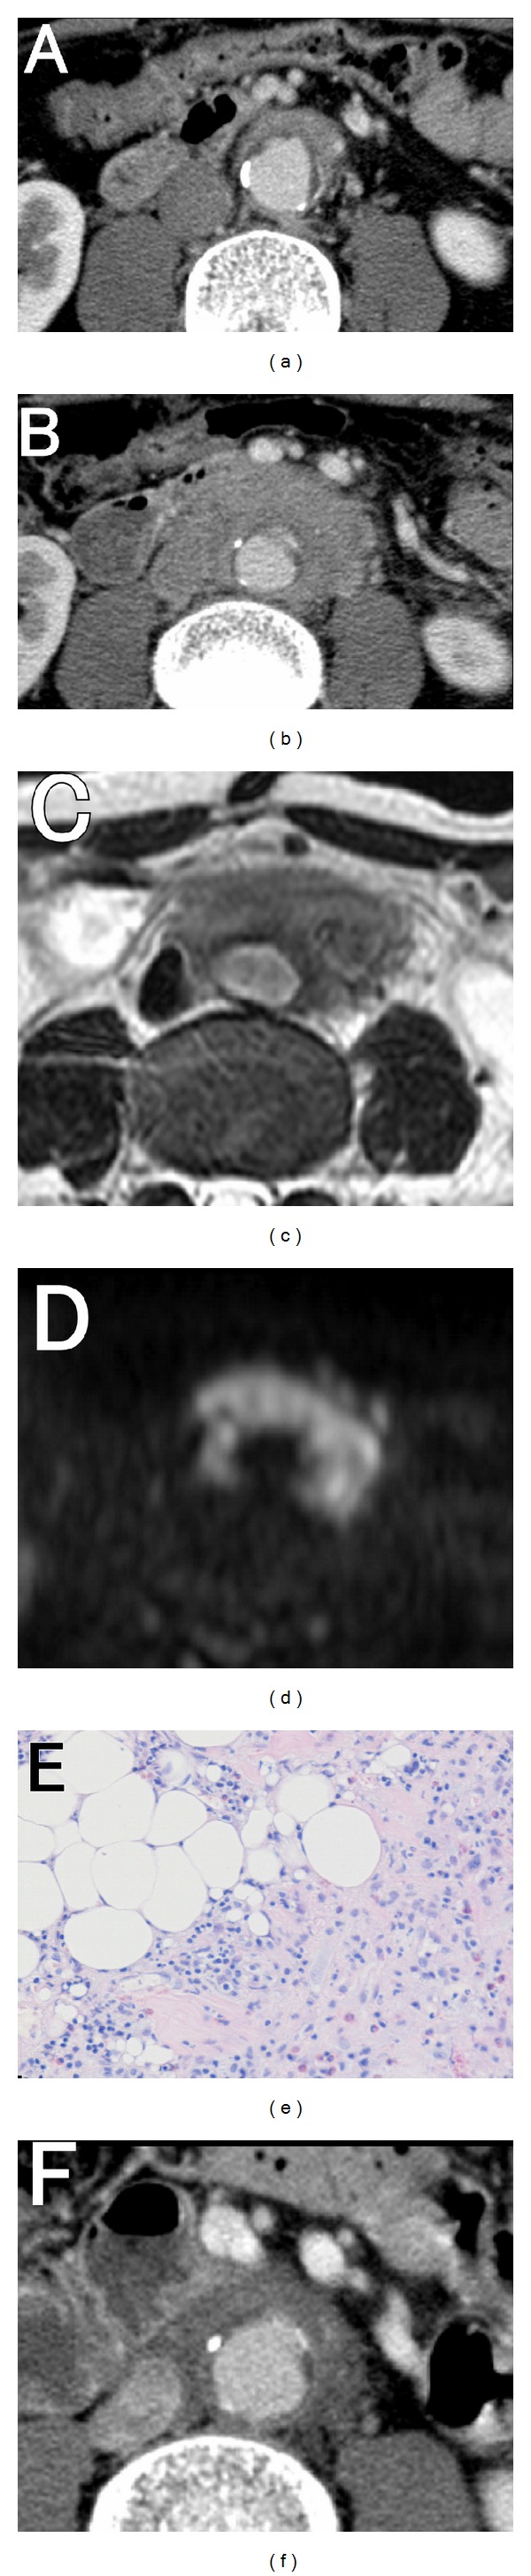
(a) A contrast-enhanced CT image, upon presentation. (b) A contrast-enhanced CT image two months after the presentation. (c) Low-intensity enhancement was observed in a T2-weighted, gadolinium-enhanced magnetic resonance image (MRI). (d) High-intensity was observed in the diffusion-weighted image. (e) A biopsy of the tissue revealed the infiltration of inflammatory cells and the absence of bacteria. (f) A contrast-enhanced CT image taken 3 weeks after starting the glucocorticoid therapy.
